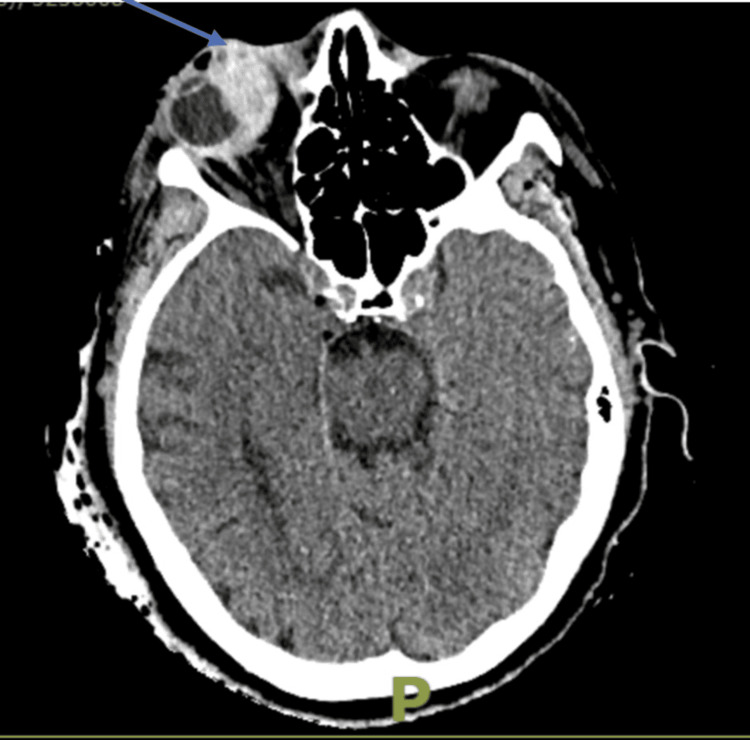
CT of brain and orbits showing a large right sided medial rectus haematoma (blue arrow) extending into the intraconal space.
